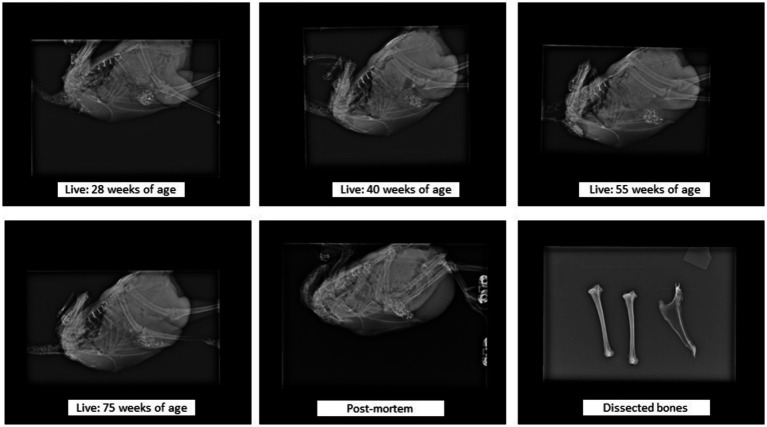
Examples of radiographic images of the same live bird (at different ages), and of the whole body. Whole-body radiograph orientations: for the body and keel bone (cranial to the left, caudal to the right, dorsal at the top of image, ventral at the bottom of image) and for the tibiotarsal bone (cranial to the bottom of image, caudal to the top of image, proximal to the left of image, distal to the right of image). Dissected bones radiograph orientations: for the keel bone (caudal to the bottom of image, cranial to the top of image, ventral margin to the left of image, dorsal margin to the right of image) and for the tibiotarsal bone (cranial to the right of image, caudal to the left of image, proximal at the top of image, distal at the bottom of image).
